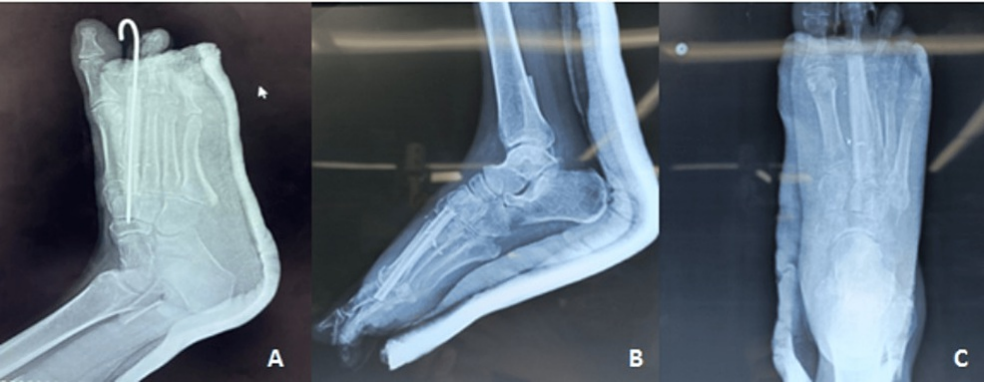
Postoperative X-ray of the foot. (A) Immediate postoperative X-ray displaying the k-wire securing the fibular implant; (B, C) X-ray taken after removal of the k-wire
